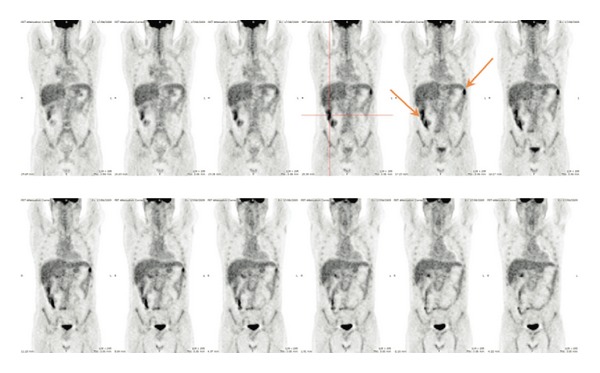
Example of a true positive patient: ^18^F-FDG PET/CT performed 25 days after allo-SCT in a 46-year-old patient who presented with signs of acute GI-GvHD 30 days after allo-SCT.
